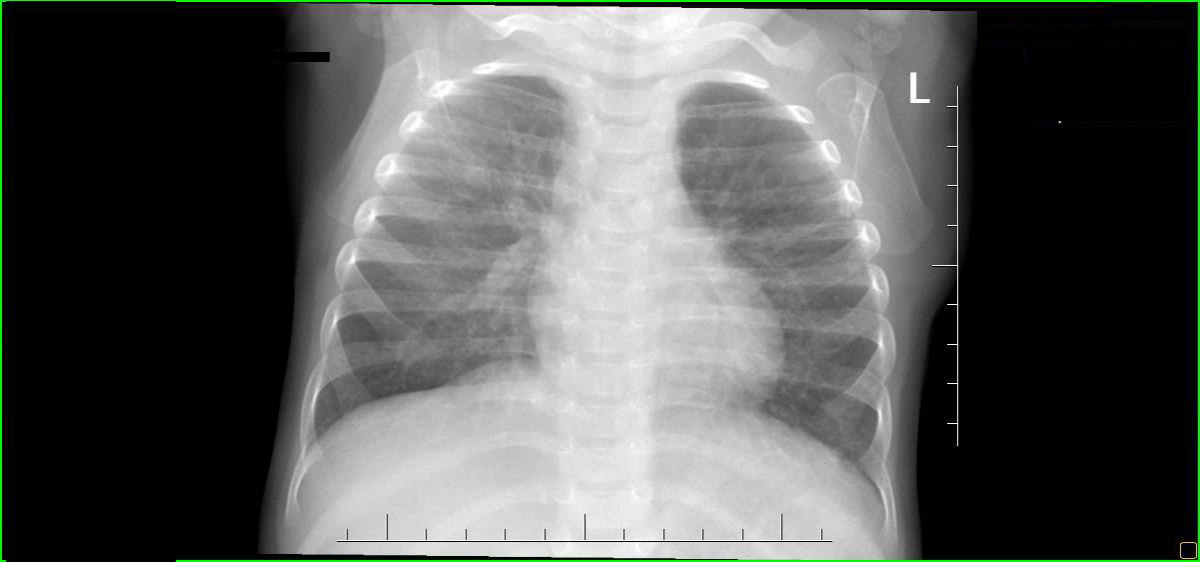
At 11 months of age, AP chest radiograph shows an improvement of lungs condition, which are mildly hyperinflated with only sparse linear interstitial markings bilaterally.
